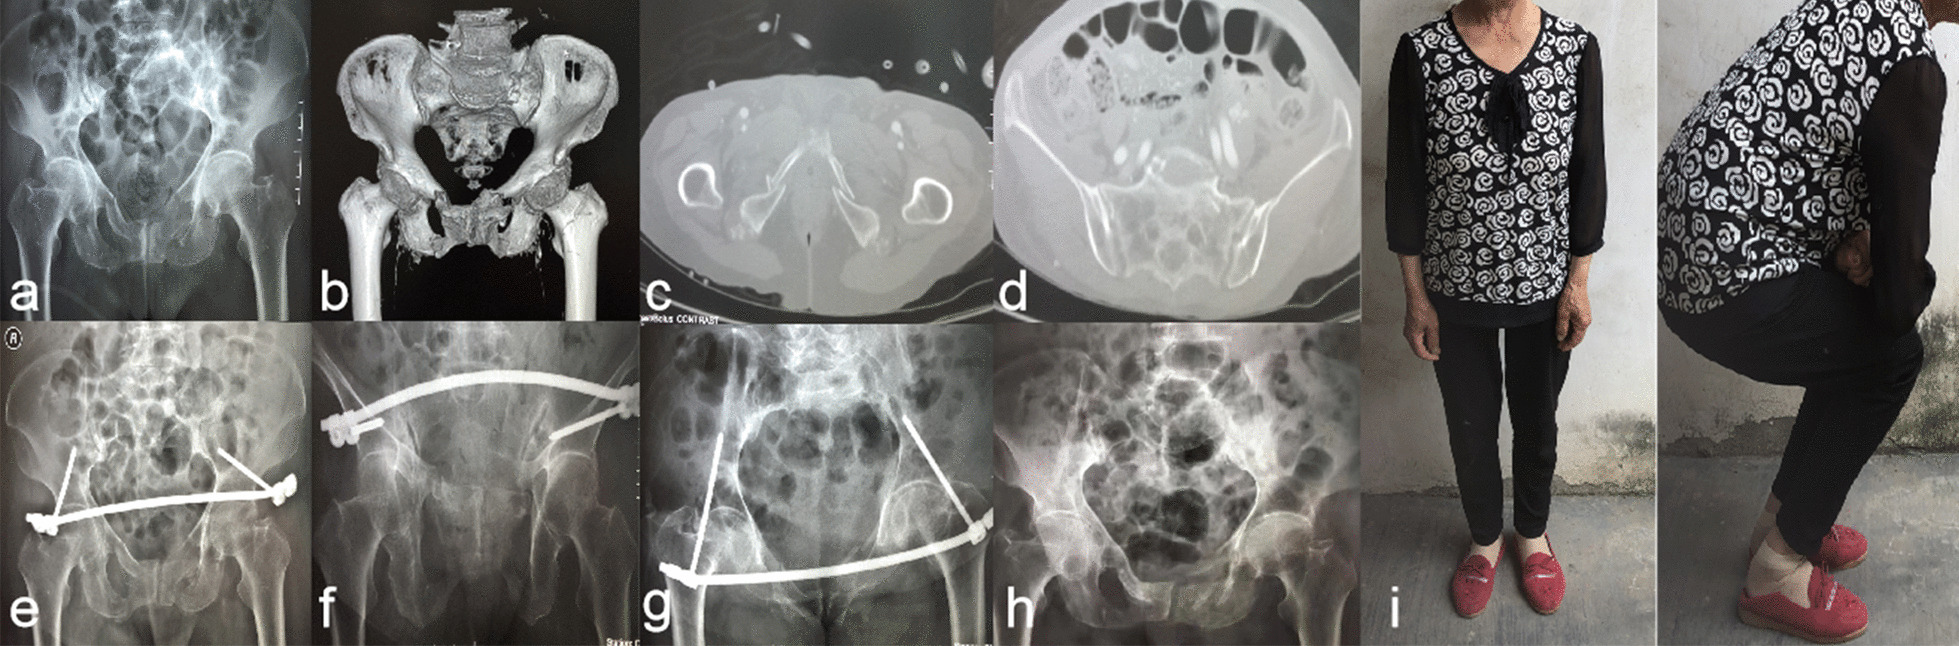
Typical case 1: A 75 years old female patient with pelvic ring fractures (AO/OTA type A) caused by a fall. The patient had a combination of gastrointestinal bleeding, moderate osteoporosis, anaemia, hypoproteinemia and diabetes mellitus. Treatment patterns of hip fractures were used pre- and post-operatively. The patient was treated with a minimally invasive closed reduction and internal fixation by the INFIX 5 days after injury. a–d Preoperative X-ray film and CT 3D reconstruction showed comminuted fractures of the upper and lower branches of the pubis. e–g The pelvic AP, outlet and inlet X rays. h Post-operative radiograph at 6 months after the INFIX stent removal, showing good fracture healing. i Post-operative functional images at 13 months
